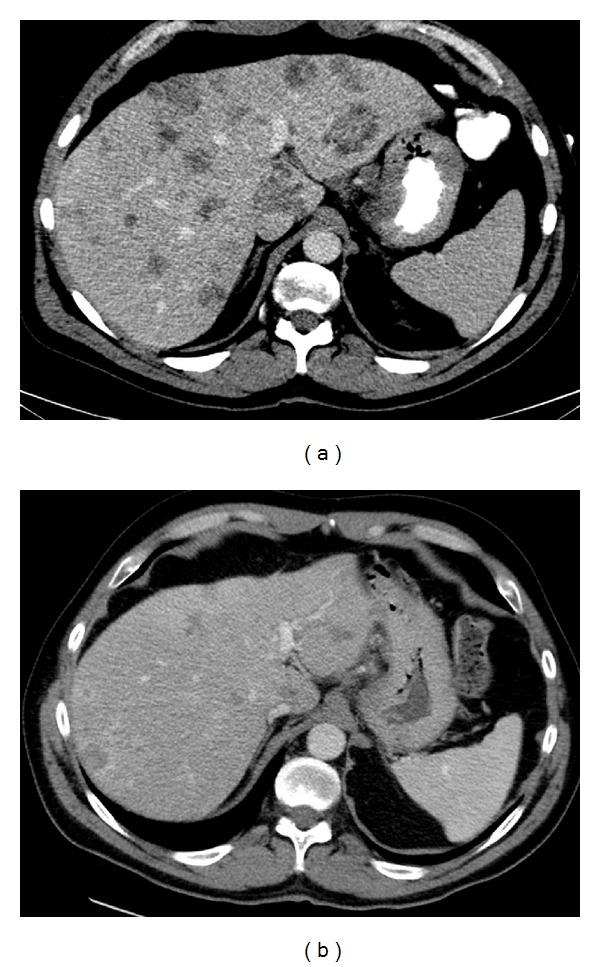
(a) Computed tomography imaging at presentation. (b) Computed tomography imaging after three cycles of cisplatin/5-FU.
